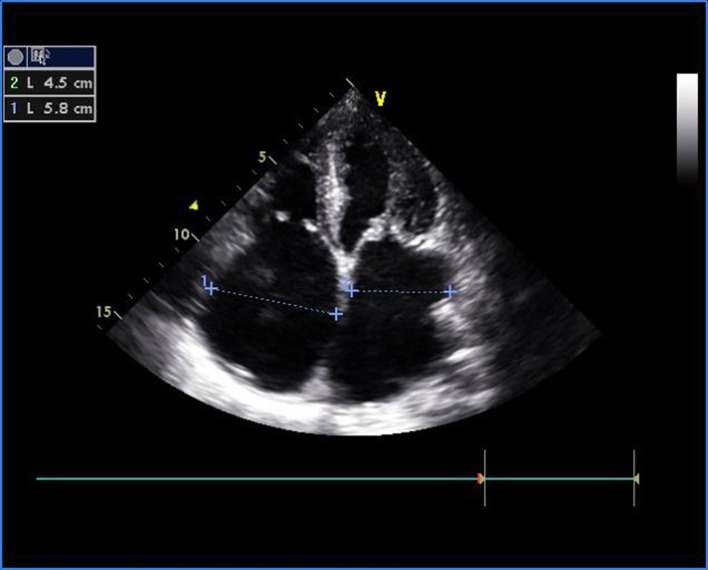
Transthoracic echocardiogram of apical four-chamber view showing thickened and doming mitral valve and tricuspid valve.
